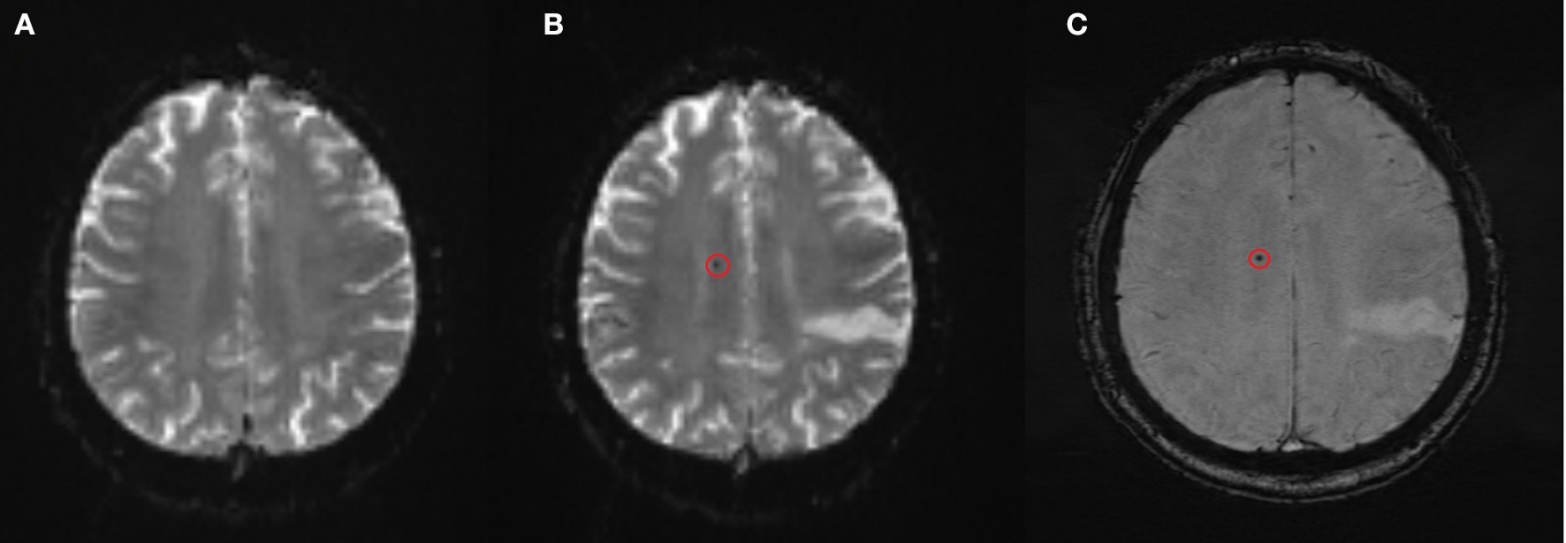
New CMB found on the follow-up MRI on day 7–9 after rtPA treatment (A) baseline T2*-weighted EPI-GRE sequence, (B) follow-up T2*-weighted EPI-GRE, (C) susceptibility-weighted imaging confirmatory slice.
